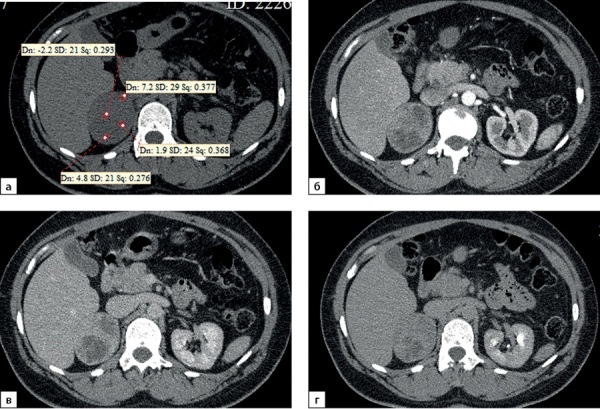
Рисунок 9. Компьютерные томограммы пациентки С., 44 лет с крупной опухолью правого надпочечника размерами (66 мм): а — изображение в аксиальной плоскости, нативная фаза сканирования; образование имеет четкие ровными контуры, умеренно диффузно неоднородную структуру с низкими денситометрическими показателями от +2,2 до +7,2 HU; б — артериальная фаза постконтрастного сканирования; в — венозная фаза; г — фаза отсроченного сканирования. Коэффициент абсолютного вымывания контрастного препарата составил 42%, относительного — 38,1%.Figure 9. Computed tomography of patient S., 44 years old, with a large tumor of the right adrenal gland (66 mm): a — image in the axial plane, native scanning phase; the formation has clear, even contours, a moderately diffusely heterogeneous structure with low densitometric values from +2.2 to +7.2 HU; b — arterial phase of post-contrast scanning; c — venous phase; d — delayed scan phase. The coefficient of absolute washout of the contrast agent was 42%, relative — 38.1%.
